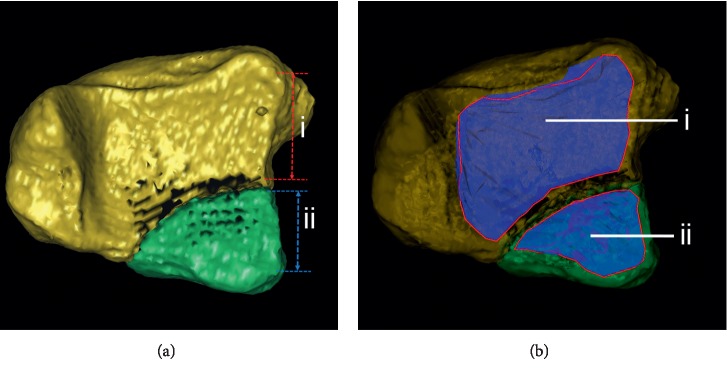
Measurement of the articular involvement of posterior malleolar fractures on 3D CT image is shown. (a) When using 3D CT linear measurement method, on the lateral border of the tibial plafond, if we assume the distance between front and back points of the residual articular surface is i and the distance between posterior malleolar fracture line and back point is ii, then the percentage of involved articular surface is ii/i + ii. (b) When using 3D CT surface measurement method, the surface boundary of the posterior malleolar fragment (ii) and residual articular surface (i) was delineated manually. Each surface area was calculated automatically by the software. Then, the ratio of the involved fragment area is ii/i + ii.
